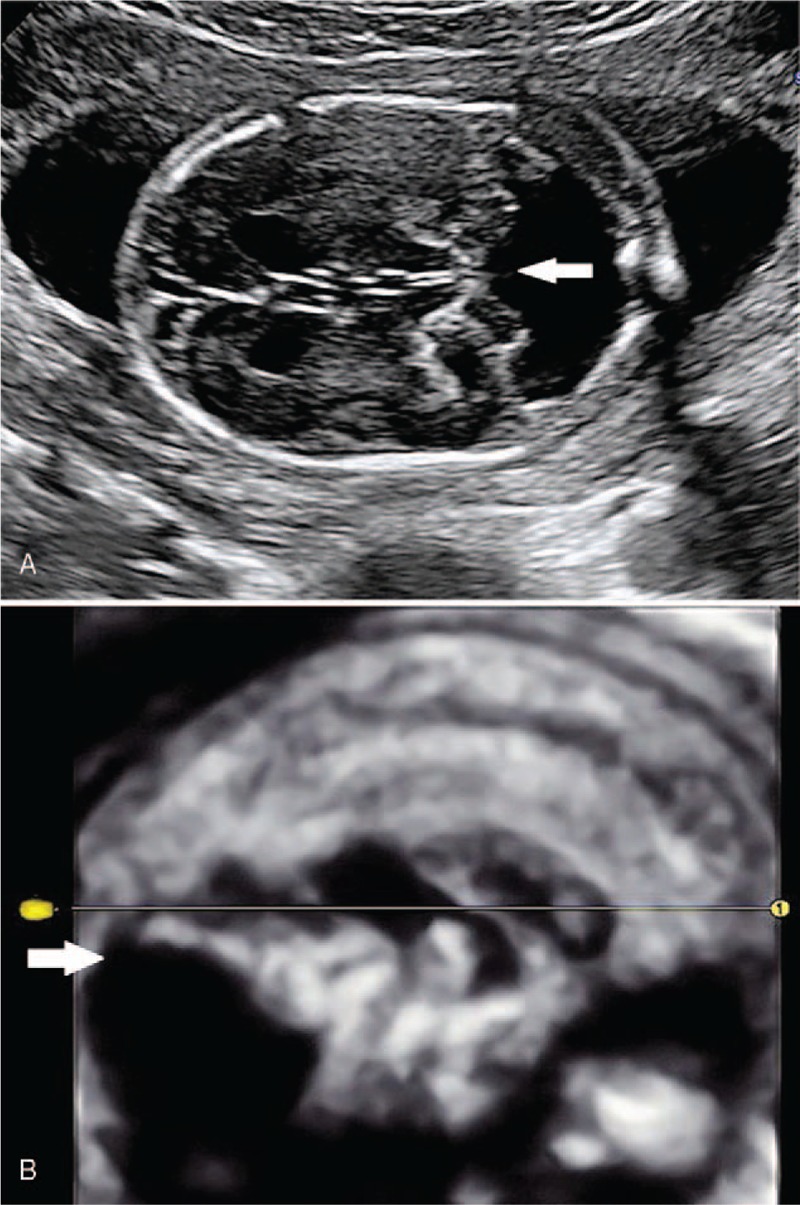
The sonographic examination of Case 1 at 21 weeks’ gestational age. (A) Transverse scan through the cerebellum showed Dandy–Walker malformation (white arrowhead). (B) 3D ultrasound rendering of the incranial structures showed the absence of cerebellar vermis and elevation of tentorium cerebelli (white arrowhead).
